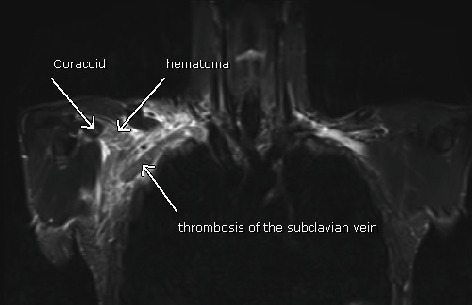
Bilateral chest wall MRI. Siemens Symphony TIM. 1.5 Tesla. Syngo MR B 19. Sequence: T2-STIR TSE coronal, 5 mm. TR 7732. TE 50. IR 140. Acquisitions Matrix: 0/320/244/0: hematoma with suspicion of axillary and subclavian vein thrombosis.
